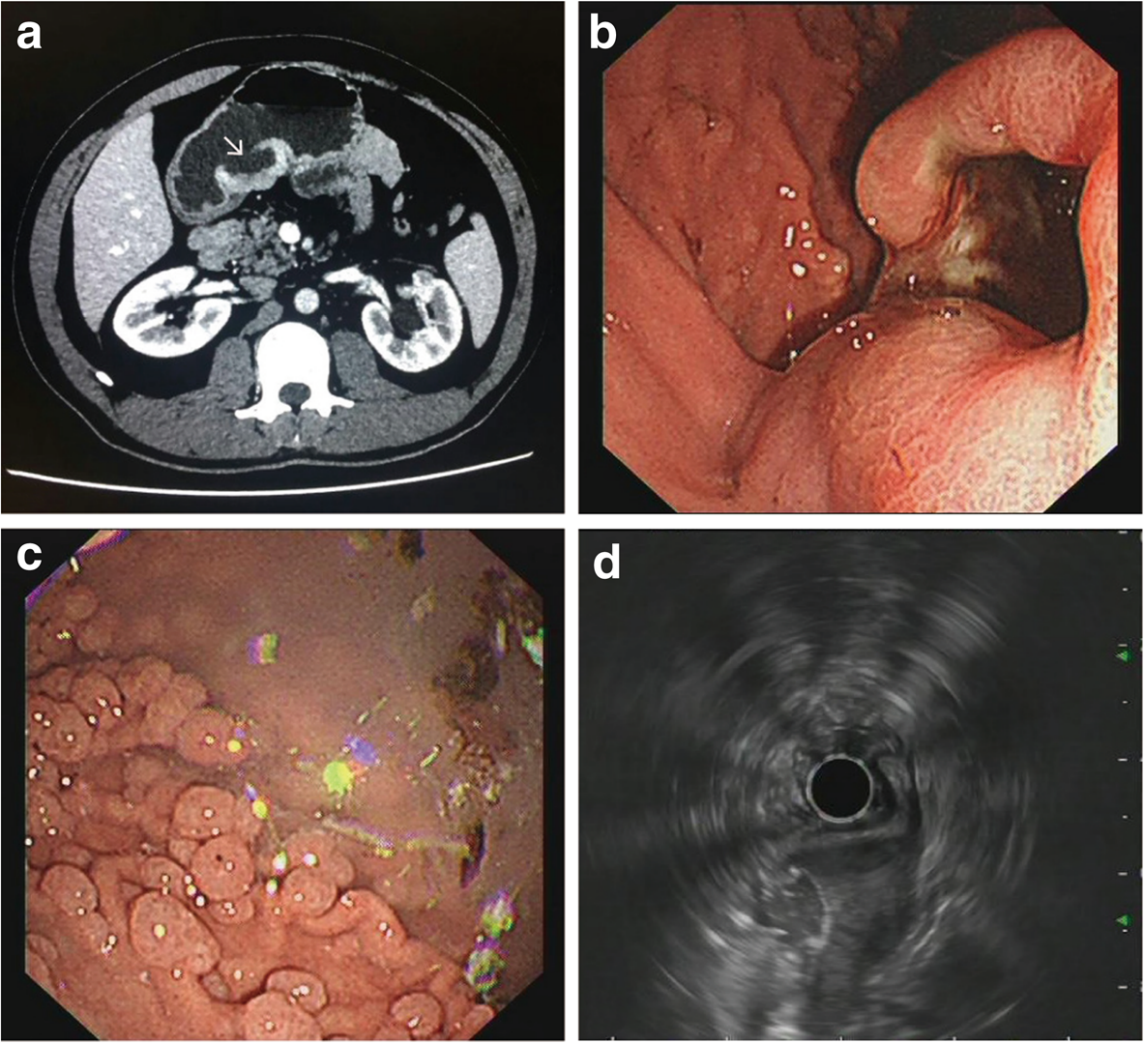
CT, endoscopy and ultrasonography examination. a Abdominal CT shows a broad-based, protruding mass with deep ulceration on the surface (*arrow*), arising from the sub-mucosal layer of the gastric antrum along the lesser curvature of the stomach. b Endoscopy shows a solid protruding mass with deep surface ulceration in the gastric angular incisures and c numerous polyps at the fundus and body of the stomach. d Electronic ultrasonic endoscopy showed the solid protruding mass
